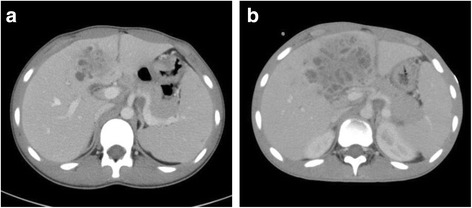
a Enhanced computed tomography (CT) image. CT image showing a multilocular mass measuring 52 mm × 34 mm in hepatic segment 4a and 5 (S4a + 5). b CT findings on day 28. The abscess has not changed since the previous scan and exhibits honeycombing
